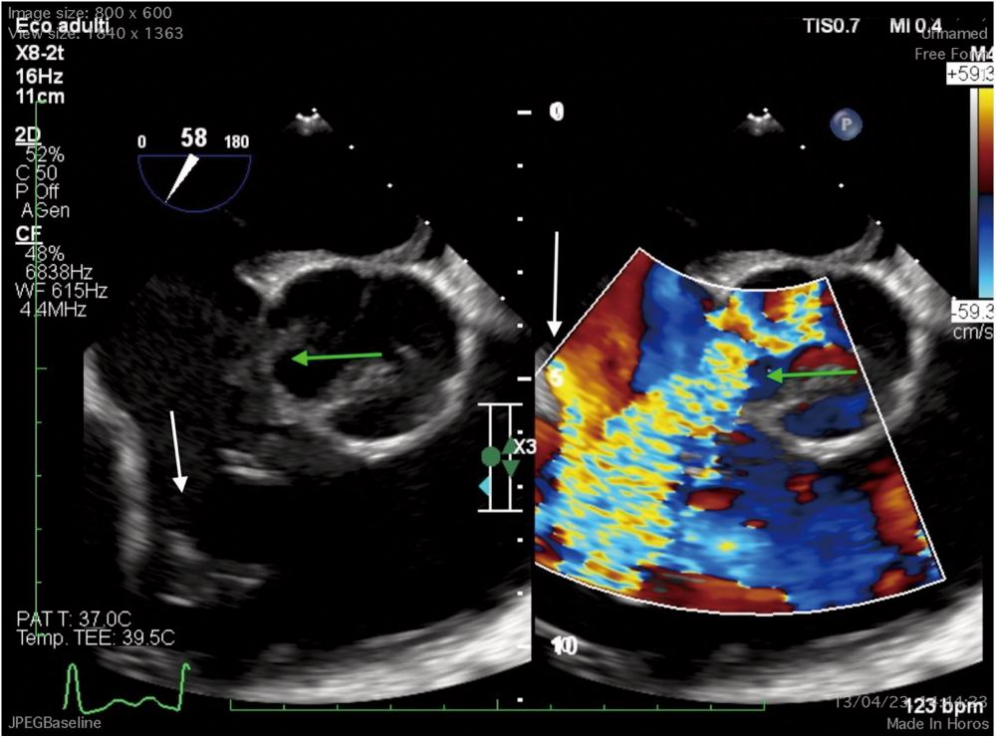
TEE (short axis), tricuspid regurgitation (white arrow) and aorta-right atrium’s shunt (green arrow) studied with colour Doppler.
